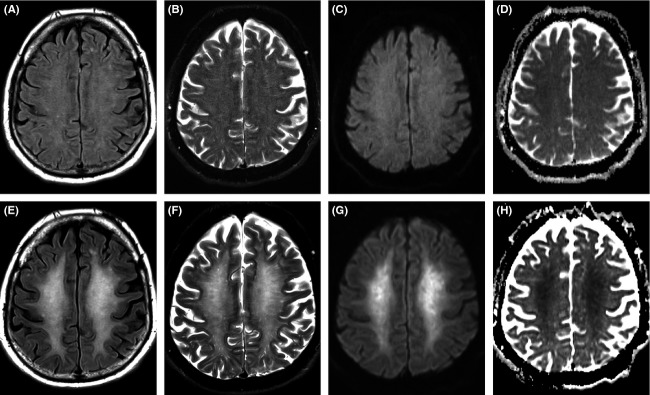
65-year-old male presenting after an acute hypoxic episode secondary to opioid and benzodiazepine overdose (case 4). Baseline MRI (A through D) demonstrate a few nonspecific subcortical T2 and FLAIR white matter hyperintensities (A and B) without restricted diffusion (C and D) probably representing chronic small vessel ischemic changes in a patient of this age. MRI after neurological relapse (E through H) shows diffuse confluent white matter abnormalities in the centrum semiovale involving the subcortical white matter. There is corresponding signal hyperintensity on DWI (G) with somewhat less extensive hypointensity on the calculated ADC maps (H), indicating some degree of T2-shine through superimposed on cytotoxic edema. FLAIR: TR = 9000, TE = 126, and IR = 2490; DWI: TR = 9000, TE = 98, number of excitations = 2, matrix = 192 × 192; field of view, 23 × 23 cm to 24 × 24 cm, slice thickness = 4 mm, and gap 4.
